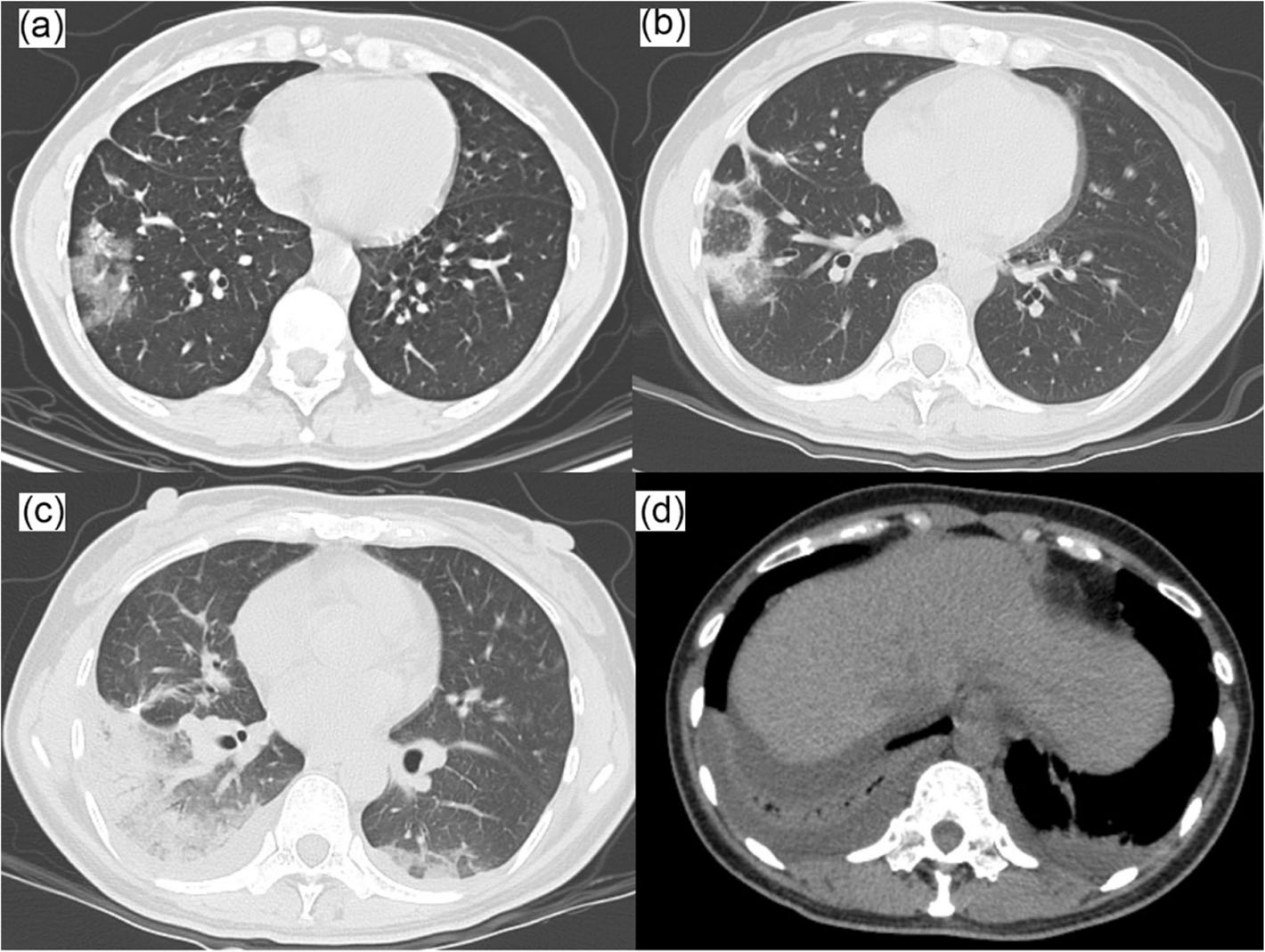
Chest CT scans. a Bilateral, blurred patches, pleural thickening and adhesions can be seen on February 7, 2020. b progressive changes with more patchy shadows on February 11, 2020. c, d Diffuse infiltrates with ground-glass opacities in the right lower lobes and bilateral Pleural effusions on February 17, 2020
